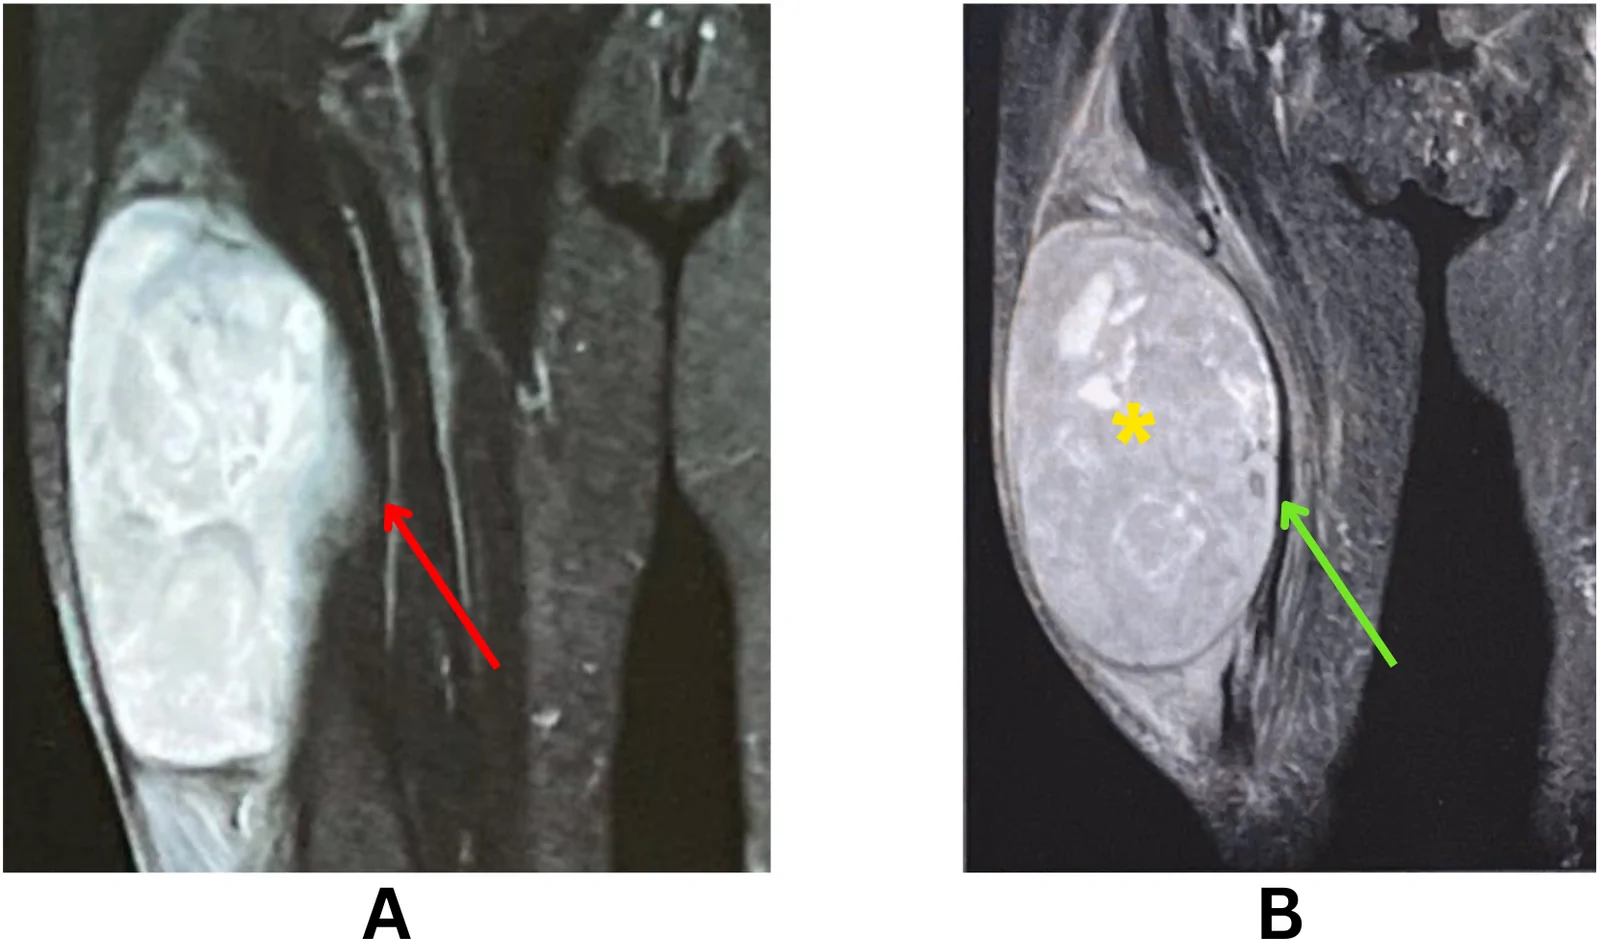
Comparative pre- and post-radiotherapy MRI (coronal T2-STIR sequences). (A) Baseline MRI: Massive soft-tissue sarcoma showing marked T2-STIR dishomogeneity with direct anatomical adherence and proximity along the femoral axis (red arrow). (B) Spatially matched follow-up MRI: 4-month post-RT evaluation demonstrating extensive intratumoral T2-hyperintensity corresponding to 95% pathologically confirmed liquefactive/coagulative necrosis (yellow asterisk). Note the appearance of a distinct low-signal fibrous demarcation plane separating the lesion from the femoral periosteum (green arrow), facilitating subsequent surgical resection. Minor visual proportion variations reflect acquisition Field-of-View (FOV) settings and treatment-induced intratumoral liquefaction/edema, while physical external tumor dimensions remained stable.
